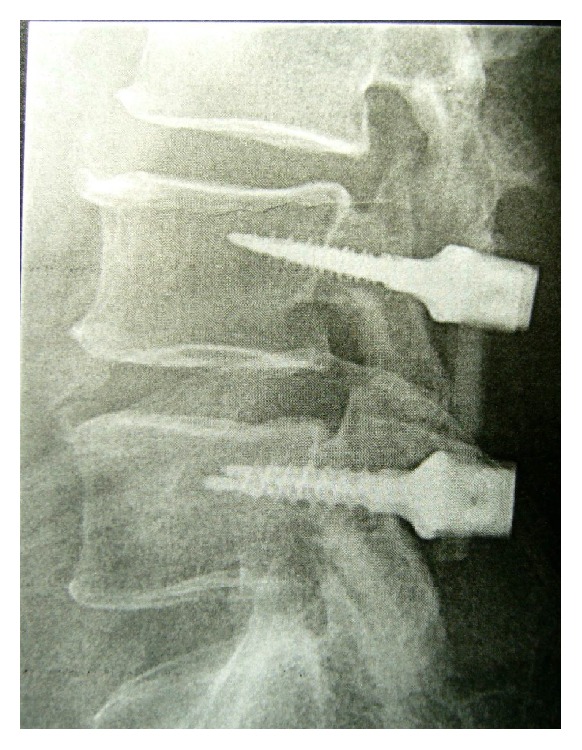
Lateral radiograph of the lumbar spine of a 74-yo patient with a canal stenosis shows spinal stability plus segmental and adjacent discs preservation.
